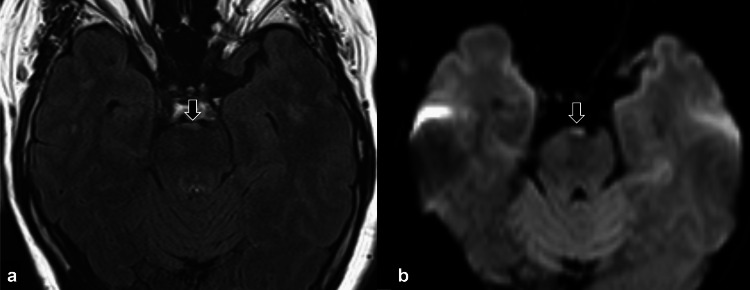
Postoperative MRI of the brain. The images show (a) axial fluid-attenuated inversion recovery (FLAIR) image and (b) axial diffusion-weighted image (DWI) (b factor: 1000 s/mm^2^). A very small amount of tumor remains near the basilar artery, which shows high signal intensity on FLAIR and DWI (a, b: arrows).
